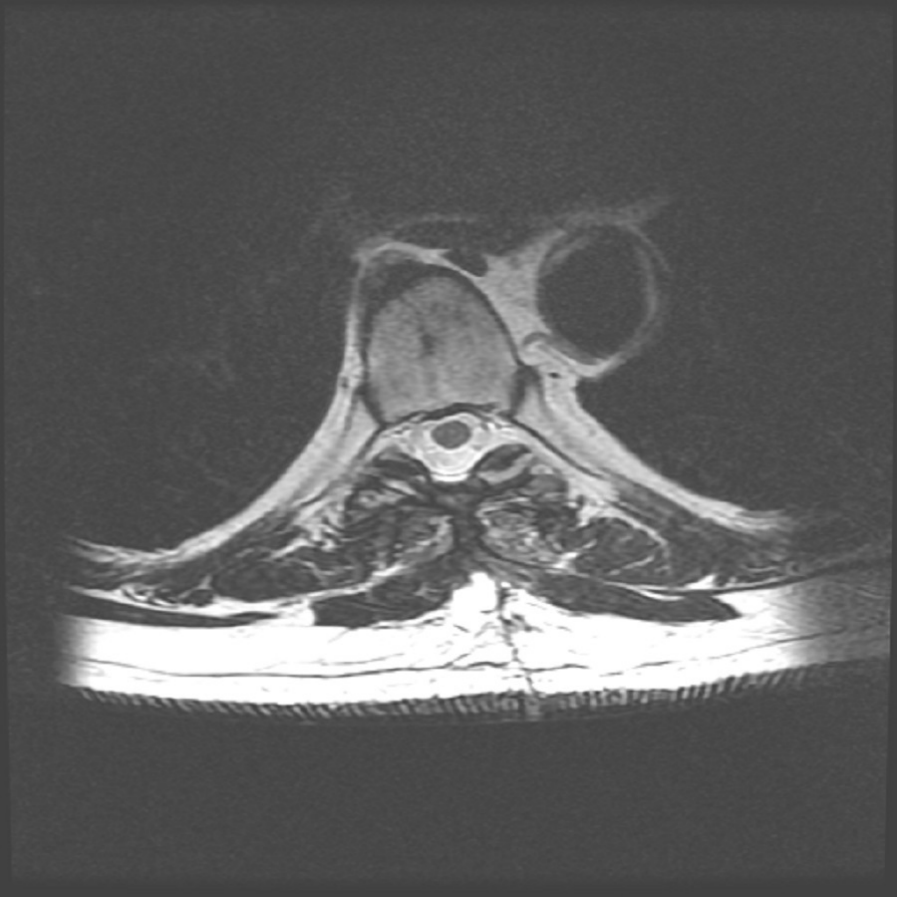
Postoperative T2 axial images. T2-weighted axial images of the thoracic spine MRI shows postoperative changes with en bloc resection of the epidural tumor with decompression of the spinal cord.
